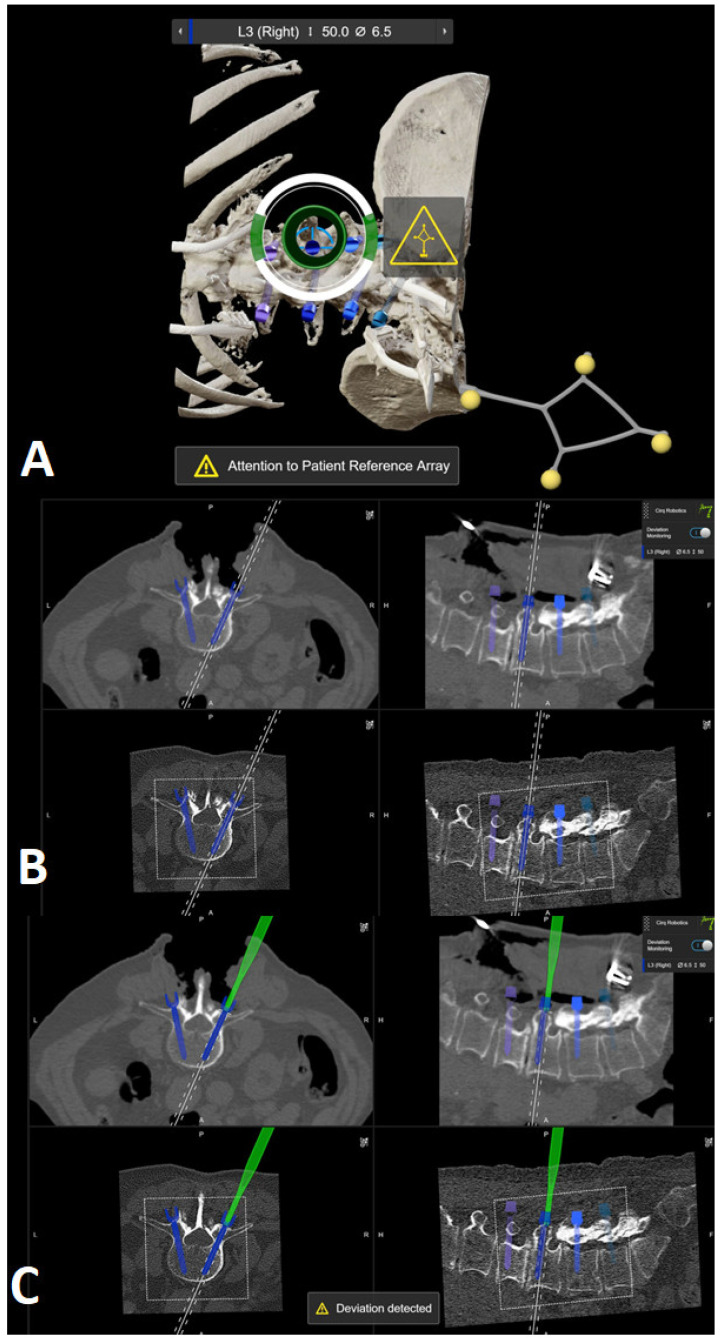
(A) Position of the robotic arm when the rough projection of the entry point of preplanned screw trajectory (in this case, the right L3 screw) is reached. The circle representing the kinematic unit of robotic arm turns green, and then the surgeon can release the robotic arm, which then automatically aligns itself according to the preplanned trajectory. (B) End position of the trajectory along the preplanned screw in the axial and sagittal view of iCT and preoperative CT. (C) Position of the drill guide instrument holder with a tracking array of the robotic arm, which can be securely locked after correct placement at the entry point. The system is very sensitive, so deviations from the original plan are reported very frequently, although these deviations are in the range of 1–2 mm.
